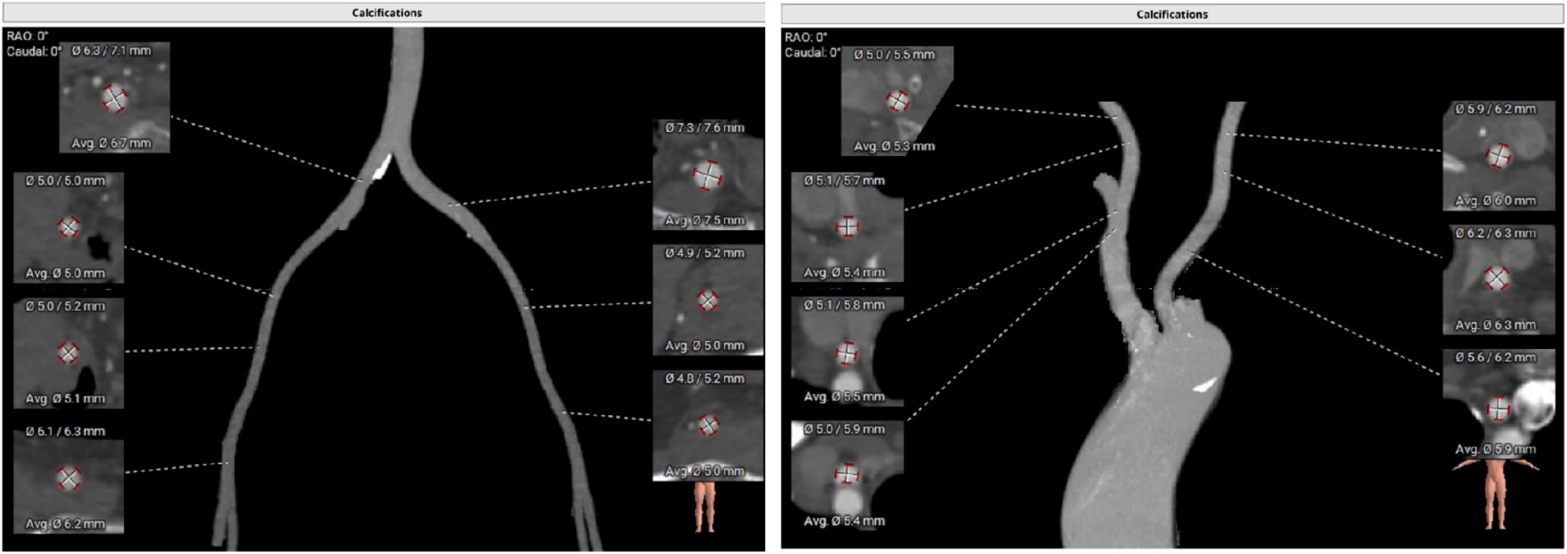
Enhanced CT examination of both common iliac arteries and carotid arteries.
